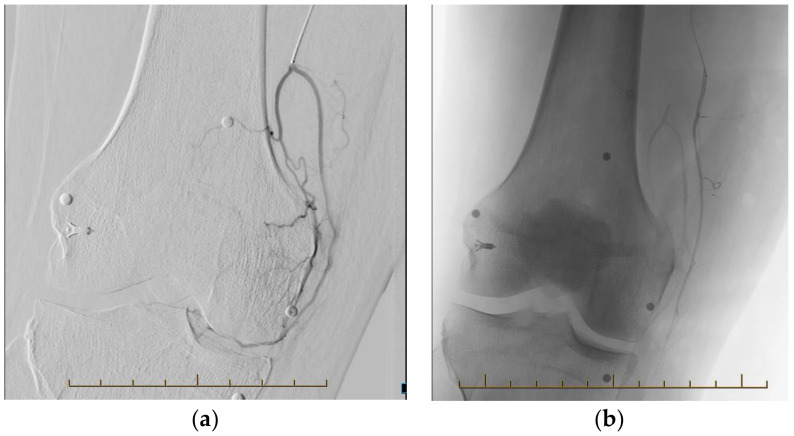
Procedural radiographs before (a) and following (b) embolization.
